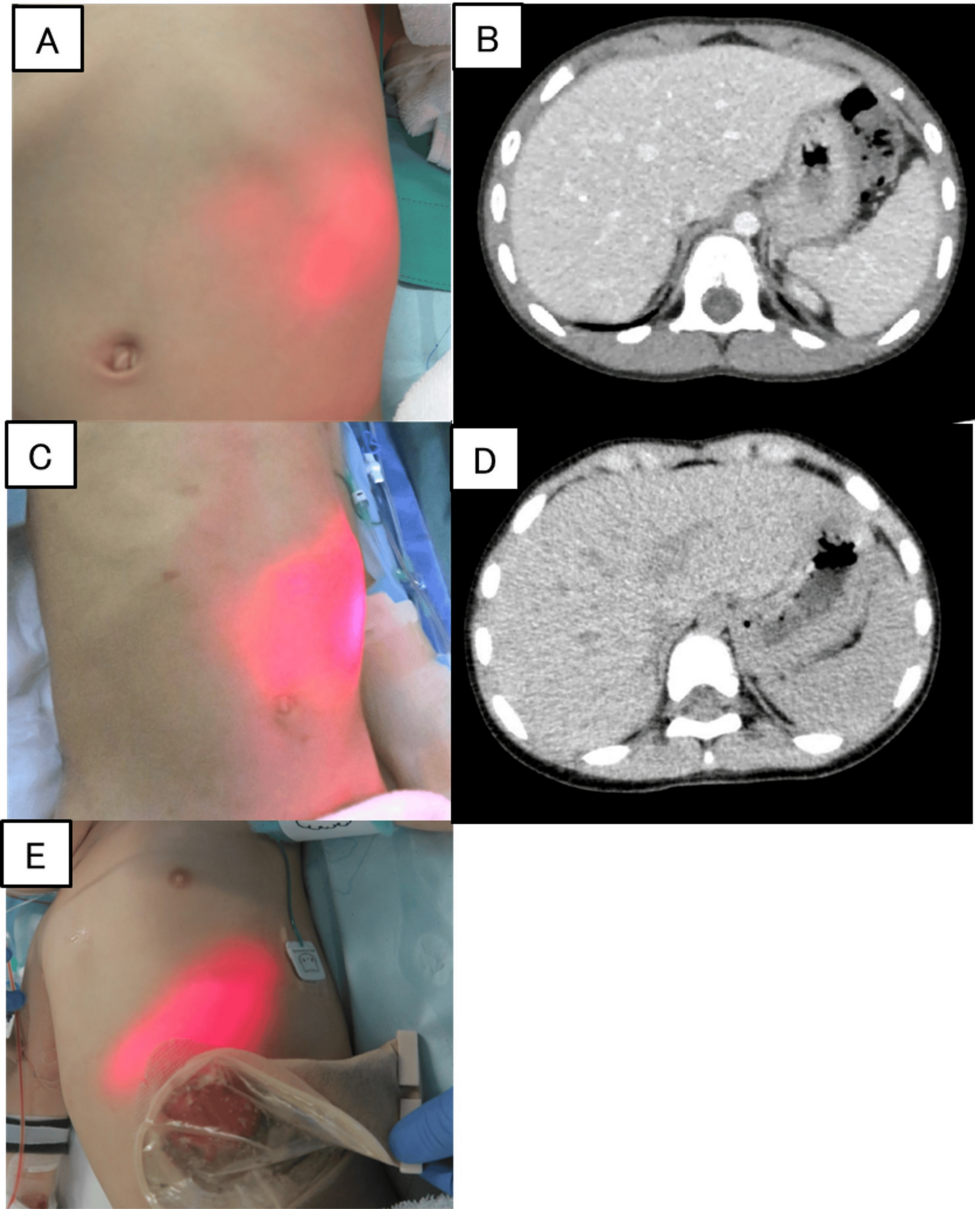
Findings in cases with other organs positioned anterior to the stomach (A-D) In cases 3 (A and B) and 5 (C and D), preoperative CT scans revealed that the left lobe of the liver was positioned anterior to the stomach. Despite this anatomical configuration, the BT light emitted from within the stomach was easily visible on the body surface, without any problems. (E) In case 2, a transverse colostomy was positioned anterior to the stomach. Despite this anatomical arrangement, the BT light emitted from within the stomach was clearly visible on the body surface without any problems BT: biologically transparent
